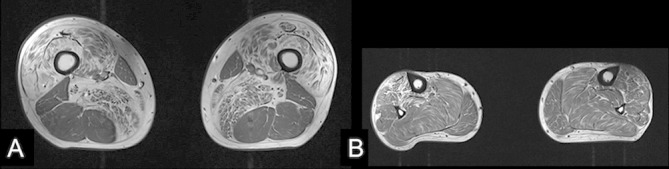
Axial T1-weighted MRI of the thigh (A) and lower leg (B) for case 1 shows fatty infiltration of the quadriceps and adductor muscles (L2, 3 and 4 myotomes; A) and tibialis anterior (L5; B) but sparing of soleus, gastrocnemius and the hamstring muscles (S1).
